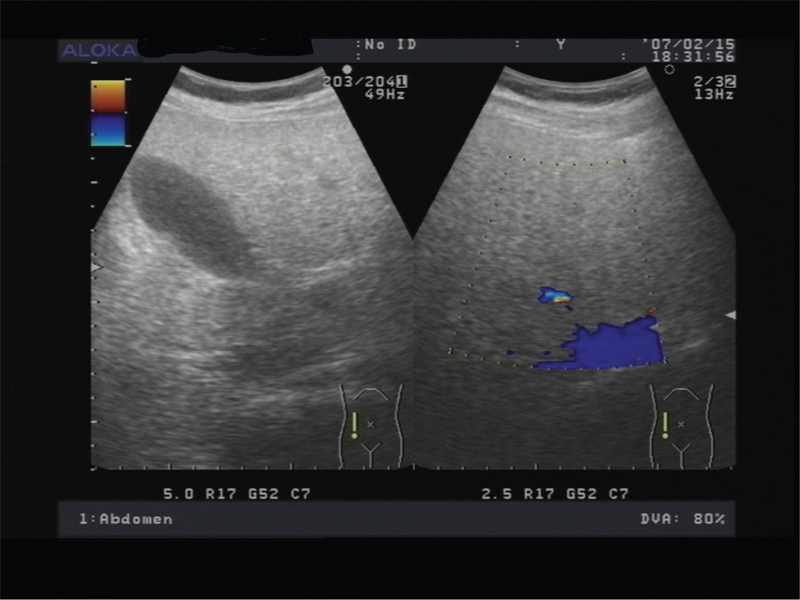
Ultrasonogram showing fatty liver. Abdominal ultrasound showed liver with an increase in echogenicity (case 1).
